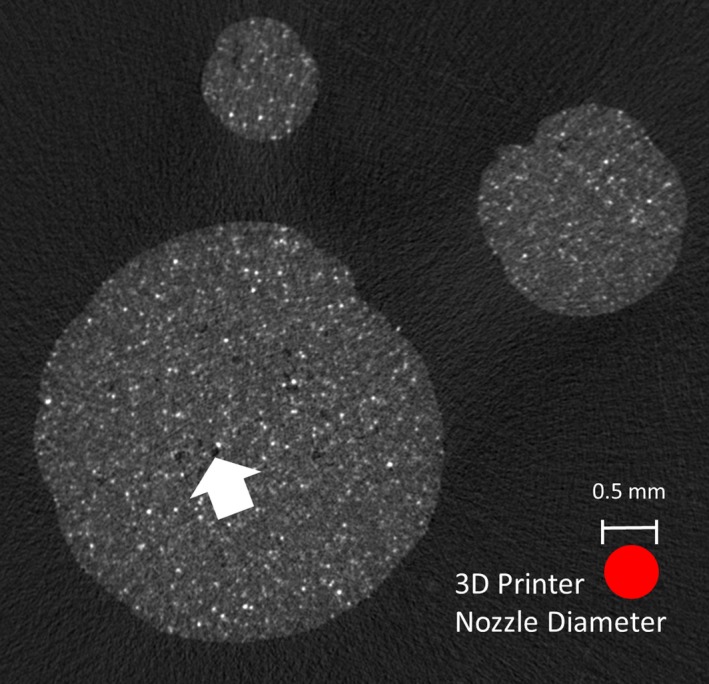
Micro‐CT image of three 3D‐printed vessel segments of varying diameters: 1.0 mm, 2.0 mm and 4.0 mm. The printer nozzle diameter was 0.5 mm, as shown. Note that small air pockets (arrow) are visible but infrequent showing that the 3D printer can reliably print and fill small‐diameter structures.
